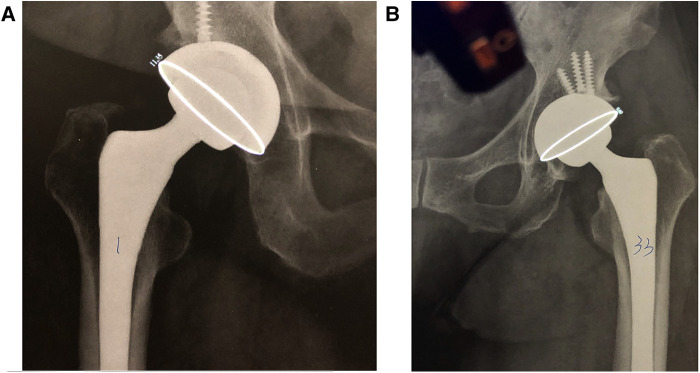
Post-operative radiographs of united total hip arthroplasty. Acetabular anteversion was measured using a portable laser projector. (A) A participant of right total hip arthroplasty with clear ellipse for measurement. With clear ellipse for the measurement, a degree of 11.35 anteversion was shown in this case following right total hip arthroplasty. (B) A participant of left total hip arthroplasty with a half of ellipse being obscured by femoral head, which is an example of poor-quality radiographs.
